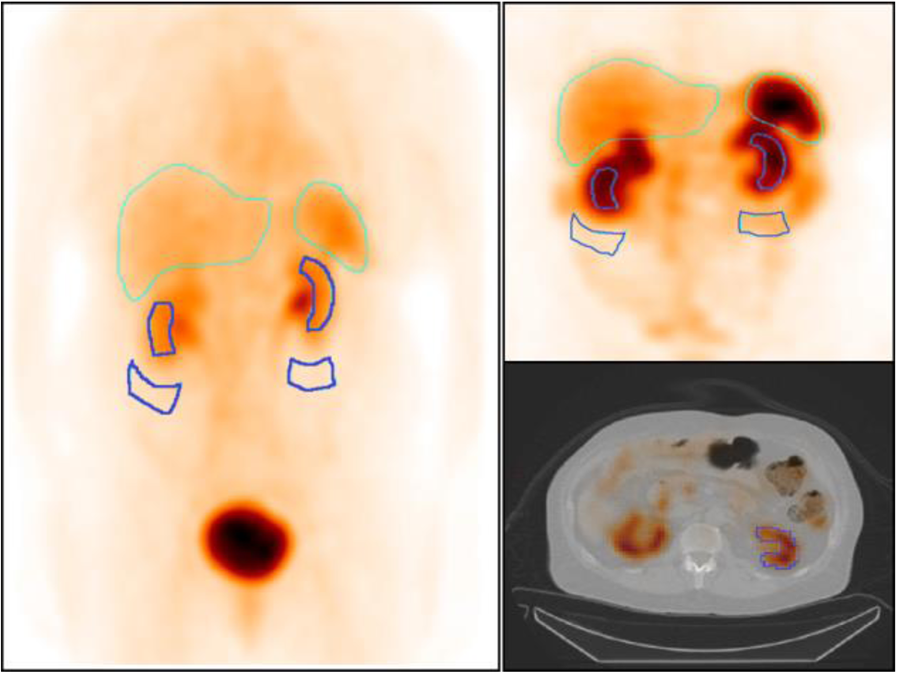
Examples of quantified planar images acquired 1 and 24 h p.i., with ROIs over kidneys and background (dark blue lines), as well as the SPECT/CT acquired 24 h p.i. with a VOI over the left kidney
